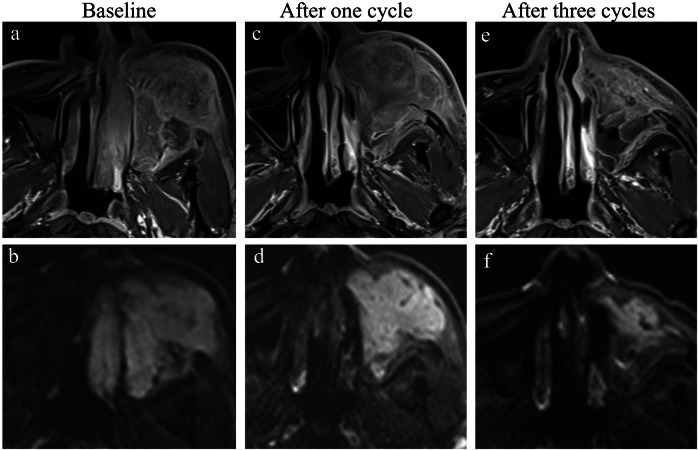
MRI findings in a patient with poorly differentiated squamous cell carcinoma in the sinonasal region, demonstrating non-pathologic complete response after three cycles of neoadjuvant chemoimmunotherapy (NCIT). a Baseline contrast-enhanced T1-weighted image (CET1WI) and (b) diffusion-weighted image (DWI) demonstrate a lesion located in the left nasal cavity and maxillary sinus region. The lesion in the nasal cavity shows a homogeneous enhancement pattern, while the lesion in the maxillary sinus region exhibits markedly heterogeneous enhancement. Follow-up CET1WI (c) and DWI (d) after one cycle of NCIT reveals complete resolution of the nasal cavity lesion, with a large residual lesion persisting in the left maxillary sinus region. e, f After three cycles of NCIT, the nasal cavity lesion remains absent, while the residual lesion in the maxillary sinus region is still present
